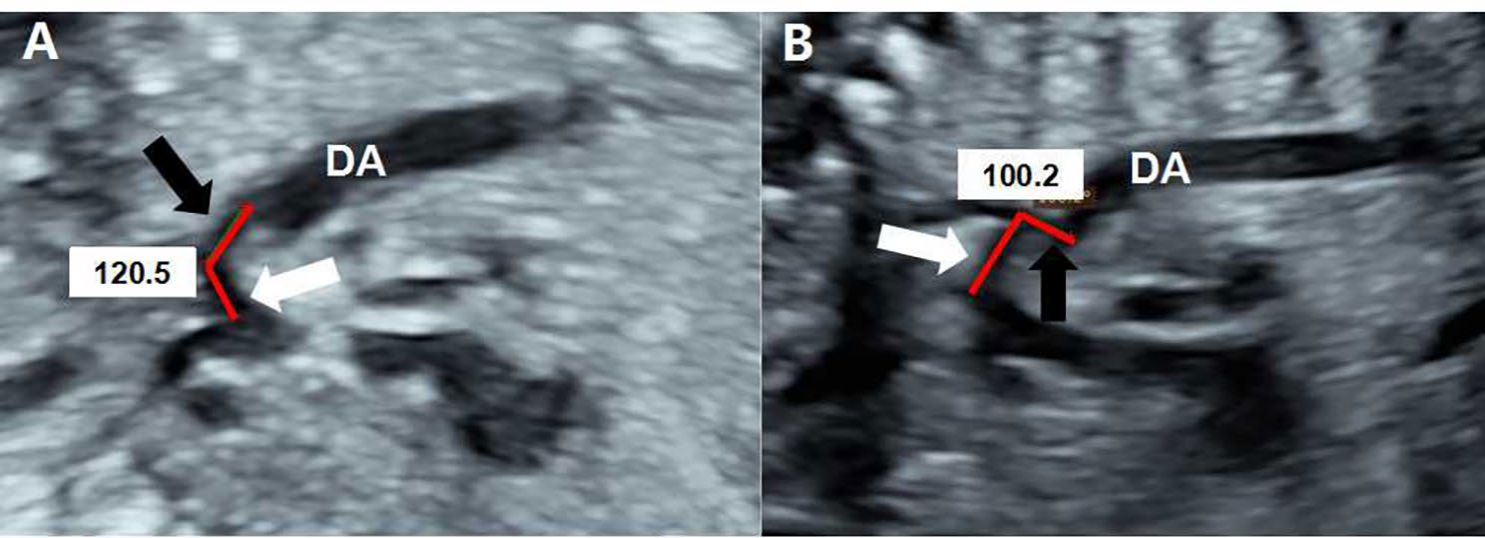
Echocardiographic images depicting the measurement of the TAO-DAO angle. In the long axis or sagittal view, one line is drawn from the origin of the left common carotid artery to the end of the left subclavian artery parallel with the transverse arch (white arrow), and the second line is drawn prior to the isthmus to the end of the isthmus (black arrow). The aortic arch has a smooth TAO-DAO angle of 120.5° in a fetus without coarctation of the aorta (A). The aortic arch presents a rigid contour with a sharp TAO-DAO angle of 100.2° in a fetus with coarctation of the aorta (B). DA, descending thoracic aorta
